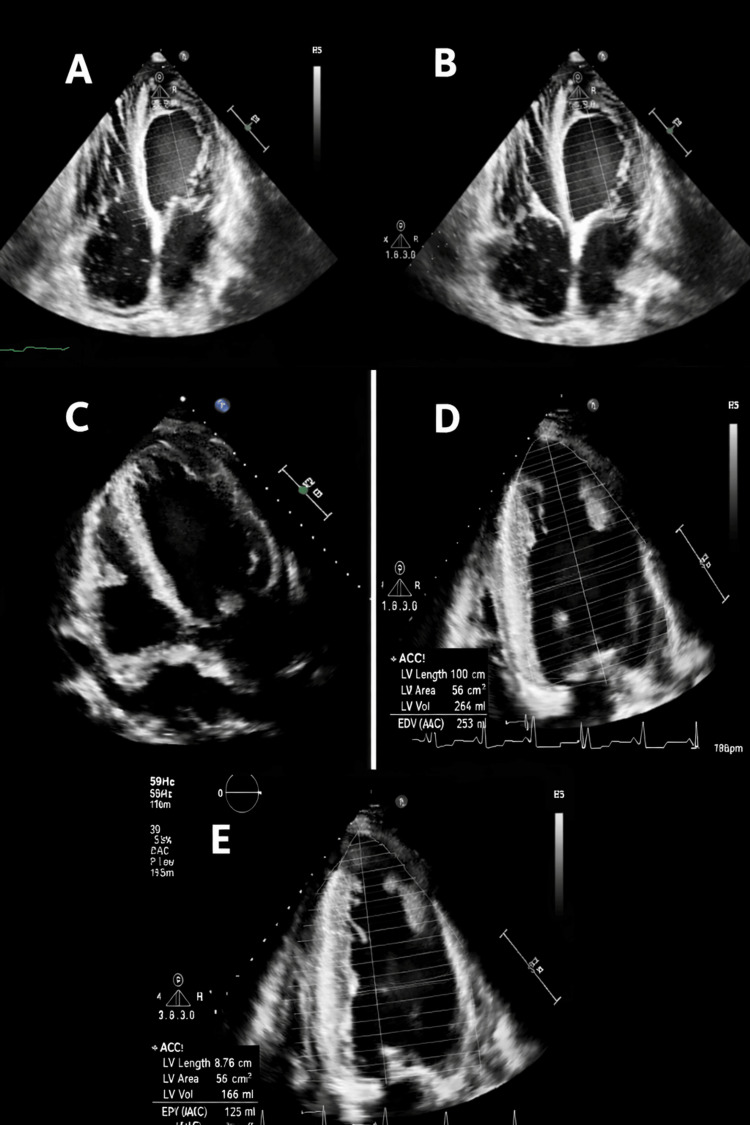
Transthoracic echocardiography (TTE) images from Case 2. (A-B) Parasternal short-axis views demonstrating prominent apical trabeculations consistent with noncompaction cardiomyopathy, along with a mild-to-moderate pericardial effusion. (C) Apical four-chamber view demonstrating a left ventricular ejection fraction (LVEF) of 35%. (D-E) Repeat TTE showing a reduced LVEF of 30.4%, indicating no significant improvement and a mild decline in systolic function.
